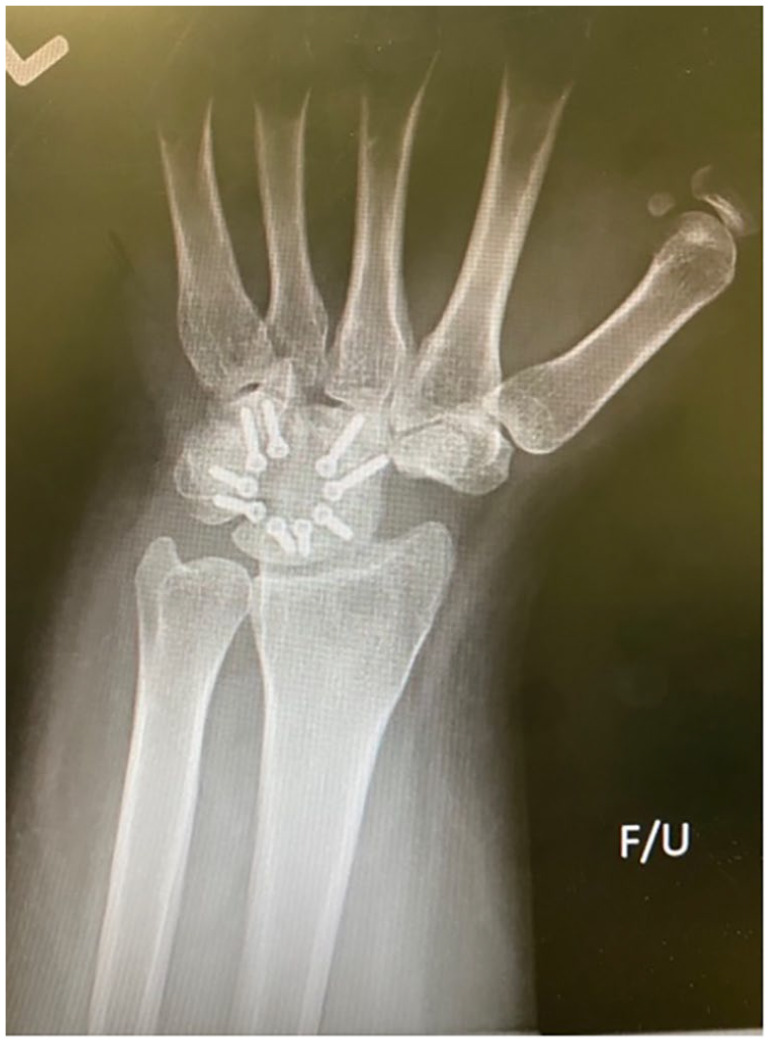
Anteroposterior radiograph of a 4-corner fusion using a circular plate for fixation of the capitate, hamate, lunate, and triquetrum. The scaphoid has been excised.
